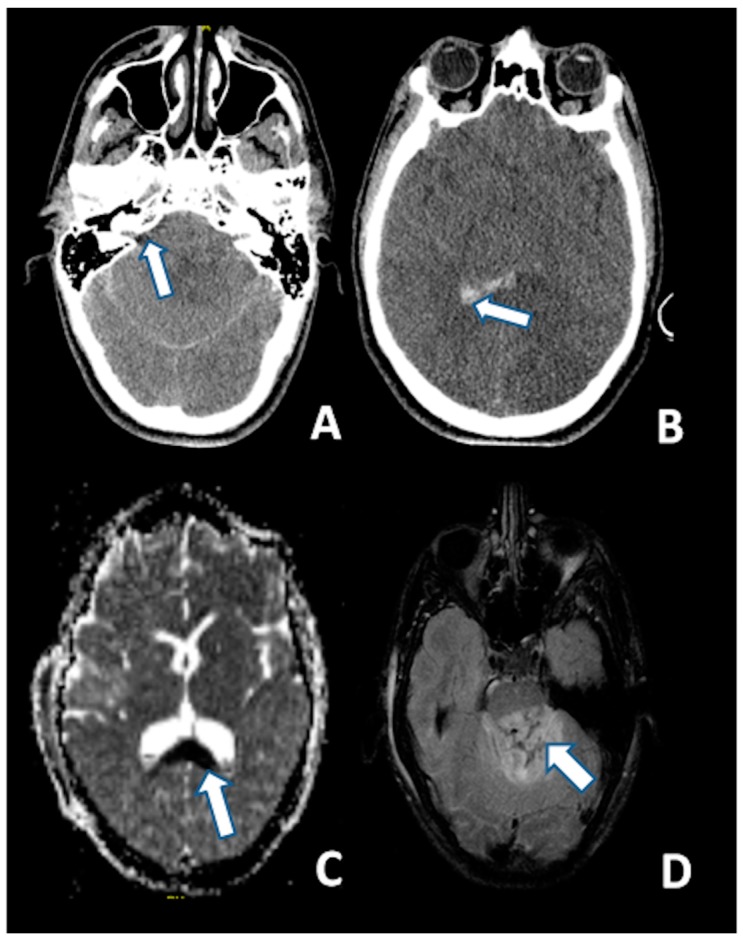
CT at 6 h after the trauma: (A) Absence of the basal cisterns (arrow); and (B) massive brain edema and intraventricular hemorrhage (arrow); MRI scan of the same patient 5 days after the trauma: (C) DWI sequence disclosing the axonal injury of the corpus callosum (arrow); and (D) injury of the dorsal aspect of the pons (arrow) (FLAIR).
